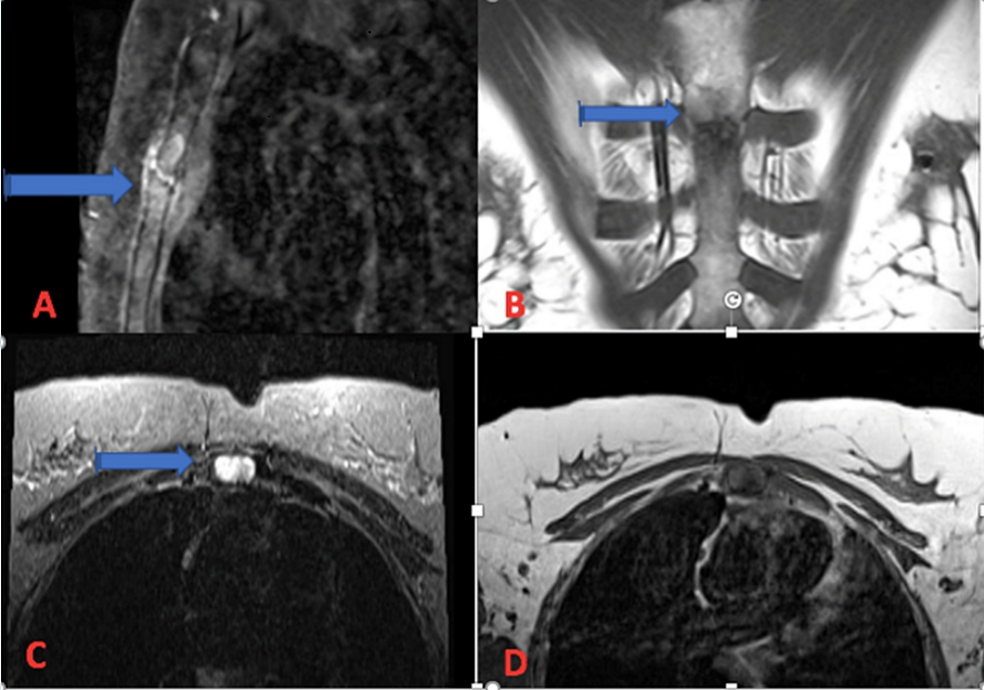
(A) Sagittal T1 fat-saturated post-contrast image of manubriosternal joint showing abnormal signal and enhancement and small erosions. (B) Coronal T1 MRI of manubriosternal joint. (C) Axial T2/STIR MRI of manubriosternal joint showing small erosions. (D) Axial T1 MRI of manubriosternal joint.
